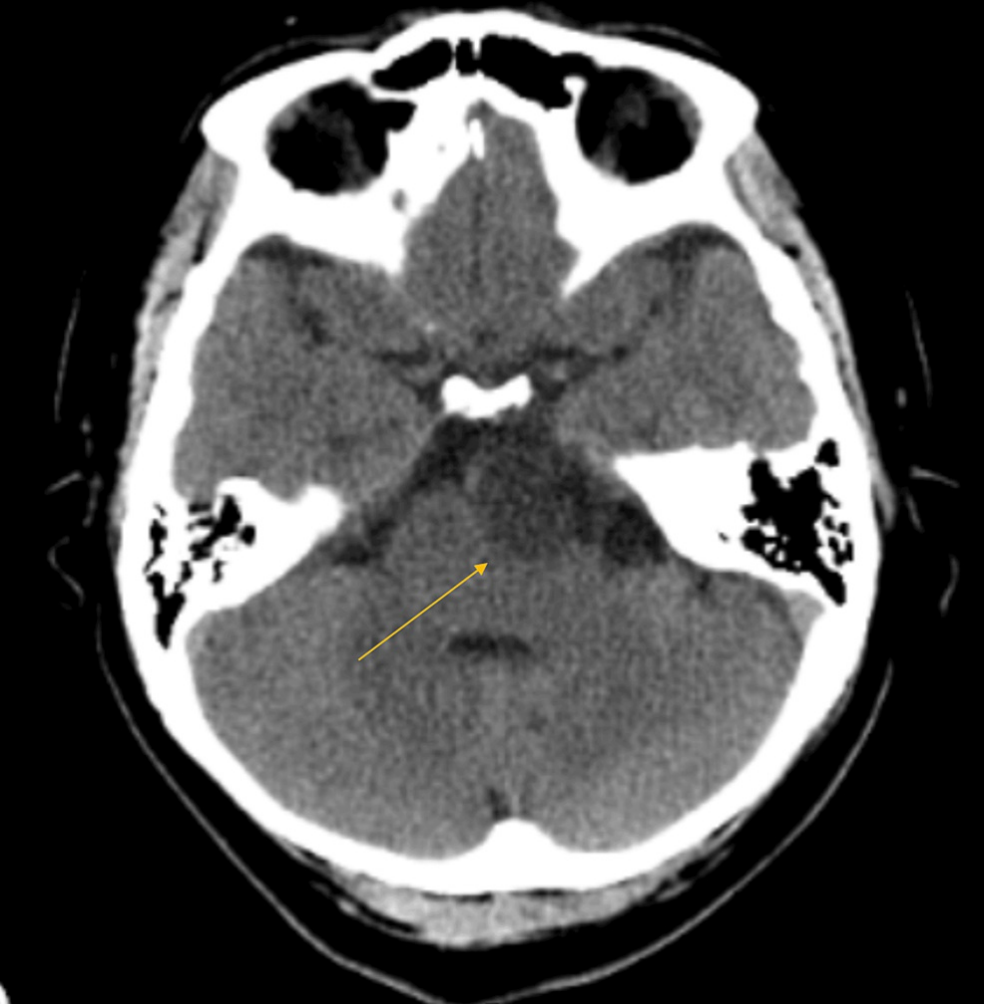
A non-contrast CT scan of the head demonstrates a cystic lesion in the left prepontine cistern with a mass effect on the left pons.
